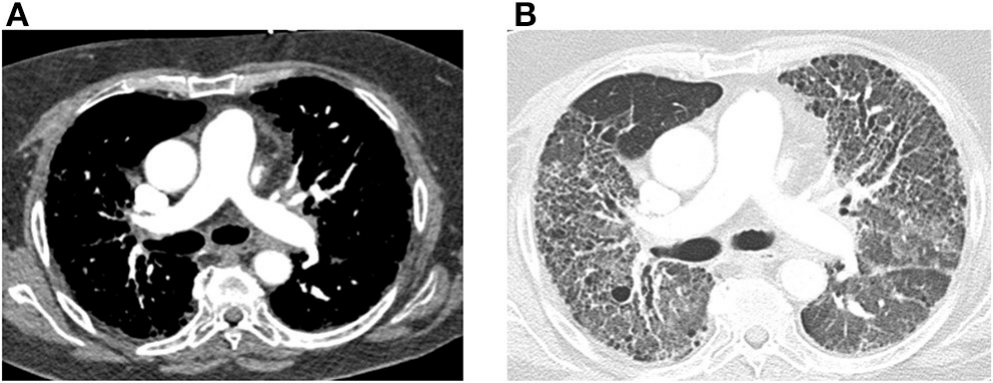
Computed tomography (CT) images of the chest with and without contrast are shown from a 64-year-old female with connective tissue disease, severe interstitial lung disease, and mixed severe pulmonary hypertension are shown. (A) Transaxial images are shown demonstrating an enlarged main pulmonary arterial size at 3.2 cm when compared to ascending aorta size of 2.9 cm at the same level suggestive of pulmonary hypertension. There is no evidence of pulmonary embolism with optimal contrast opacification. (B) Transaxial images in the lung window demonstrate extensive bilateral diffuse groundglass opacities and honeycombing. There is associated intralobular and interstitial thickening and bronchiectasis consistent with patient's known history of connective tissue disease associated non-specific interstitial pneumonitis.
